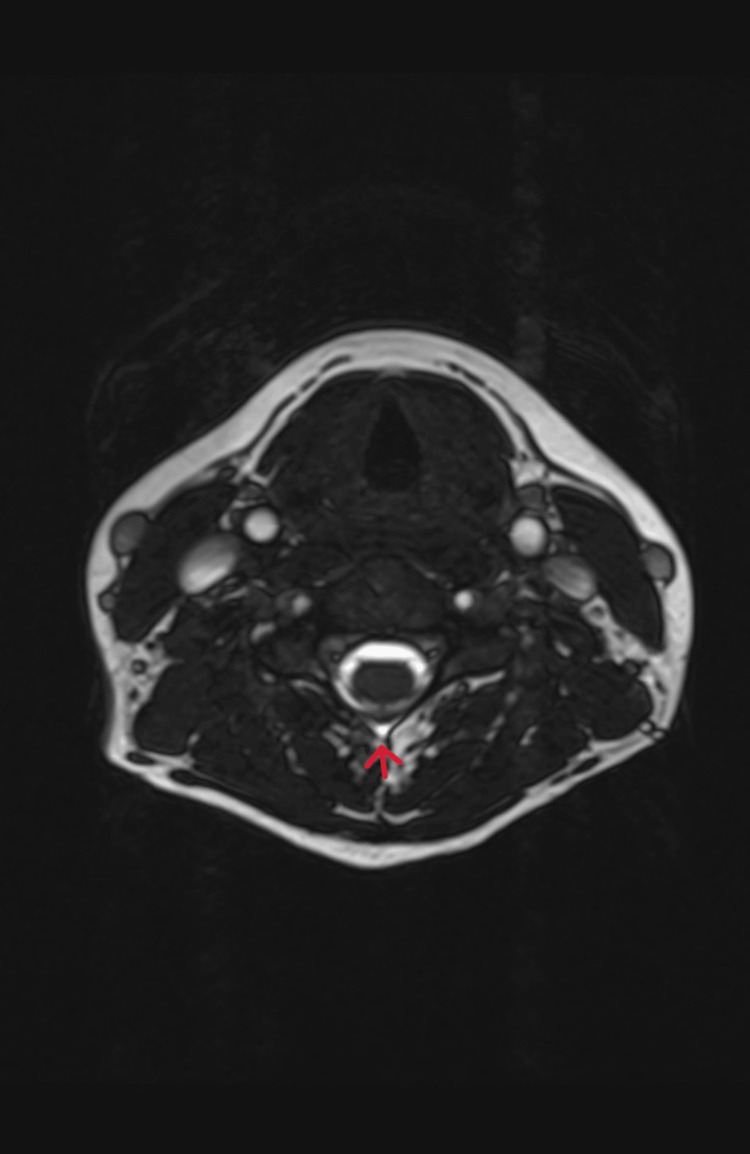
MRI constructive interference in steady-state sequence axial view showing fluid signal at the level of the C4/C5 interspinous process.
